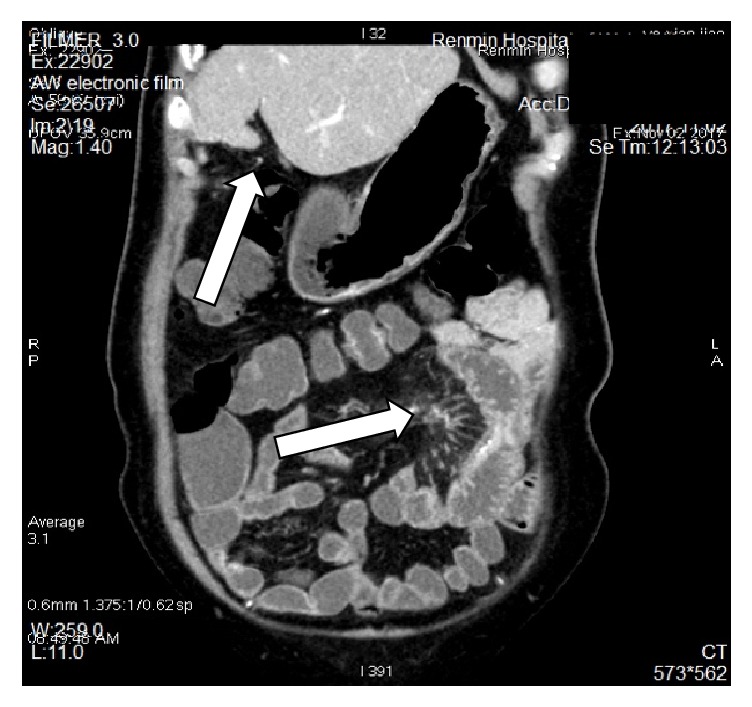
CT scan showing atrophy of the right liver lobe with hypertrophy of the left and caudate lobes, mesenteric edema.
